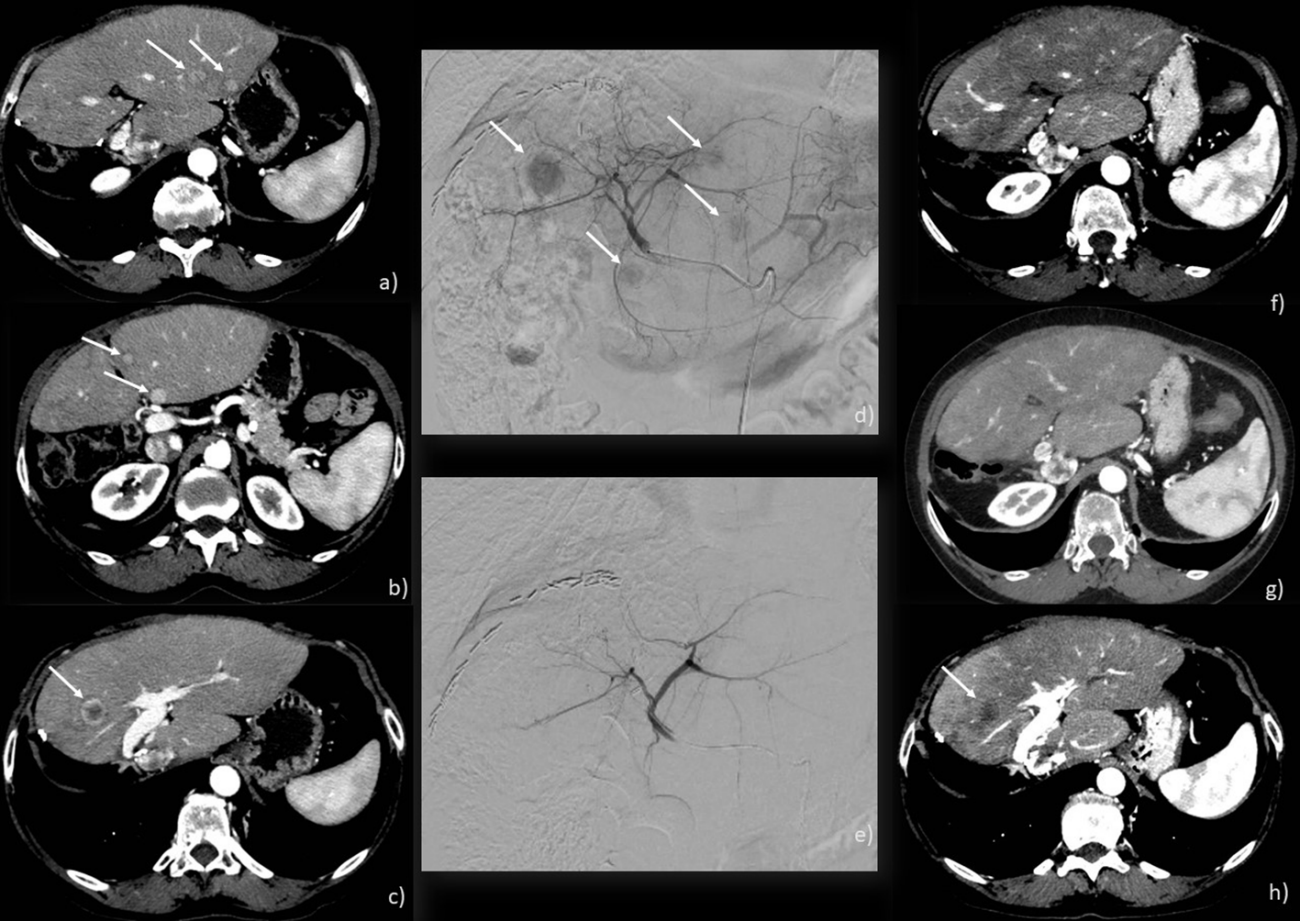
c-TACE performed on a 71-year-old woman: (A–C) CT scan showing 4 ICC nodules in both liver lobes (arrows); (D) angiographic study showing ICC nodules (arrows); (E) angiographic study after injection of chemotherapeutic showing the exclusion of the feeding vessels to the lesions; (F–H) CT scan post-treatment showing a good response with necrosis of the main lesion (arrow) and no new emerging lesions.
